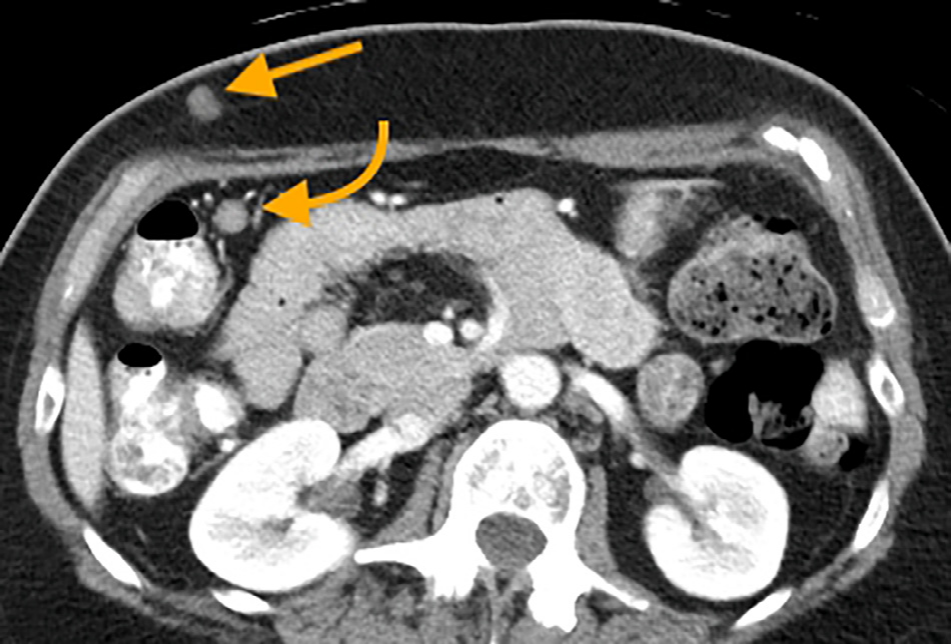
CT demonstrating a subcutaneous nodule in the right abdominal wall (straight arrow) and a prominent mesenteric lymph node in the right abdomen (curved arrow).
